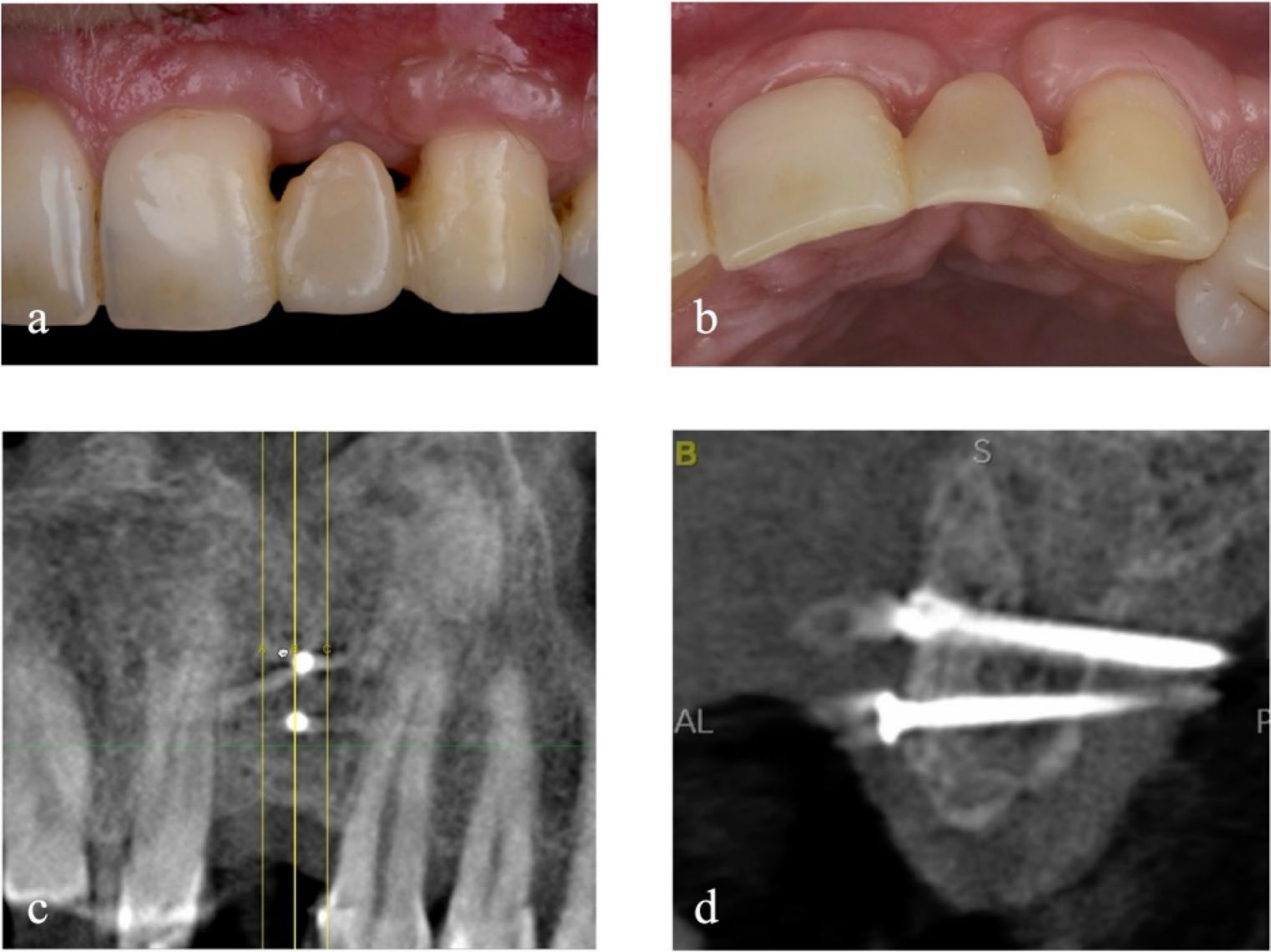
Detail of soft tissue (a, b) and CBCT examination of the reconstructed refect (c, d) 6 months after grafting procedure
